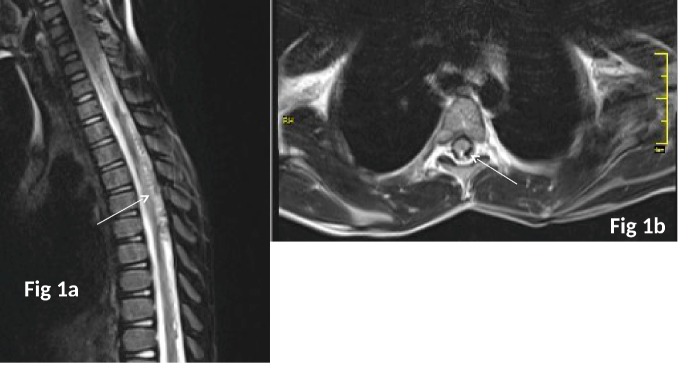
Figure 1: Sagittal T2 Weighted Images (Figure 1a) of Spine showing abnormal flow voids (white arrow) posterior to spinal cord in cervico-dorsal spine (C6-D4). MRI Spine (T2 Weighted axial Images- Figure 1b) showing abnormal flow voids in cervico-dorsal spine (C6-D4) with intramedullary bleed (white arrow).
